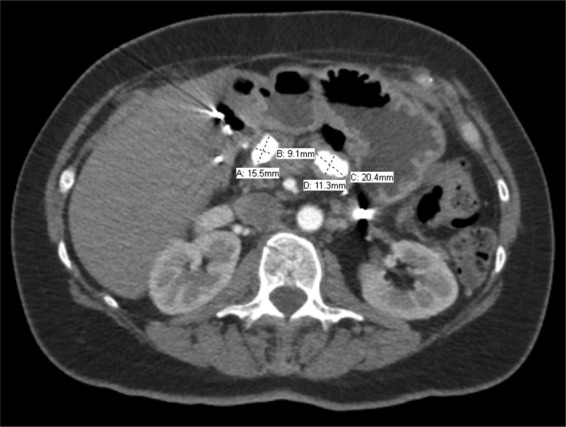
Computed tomography image showing two substantial calcifications in the pancreatic remnant and their measured size. Note the absence of the spleen in the left upper quadrant.
